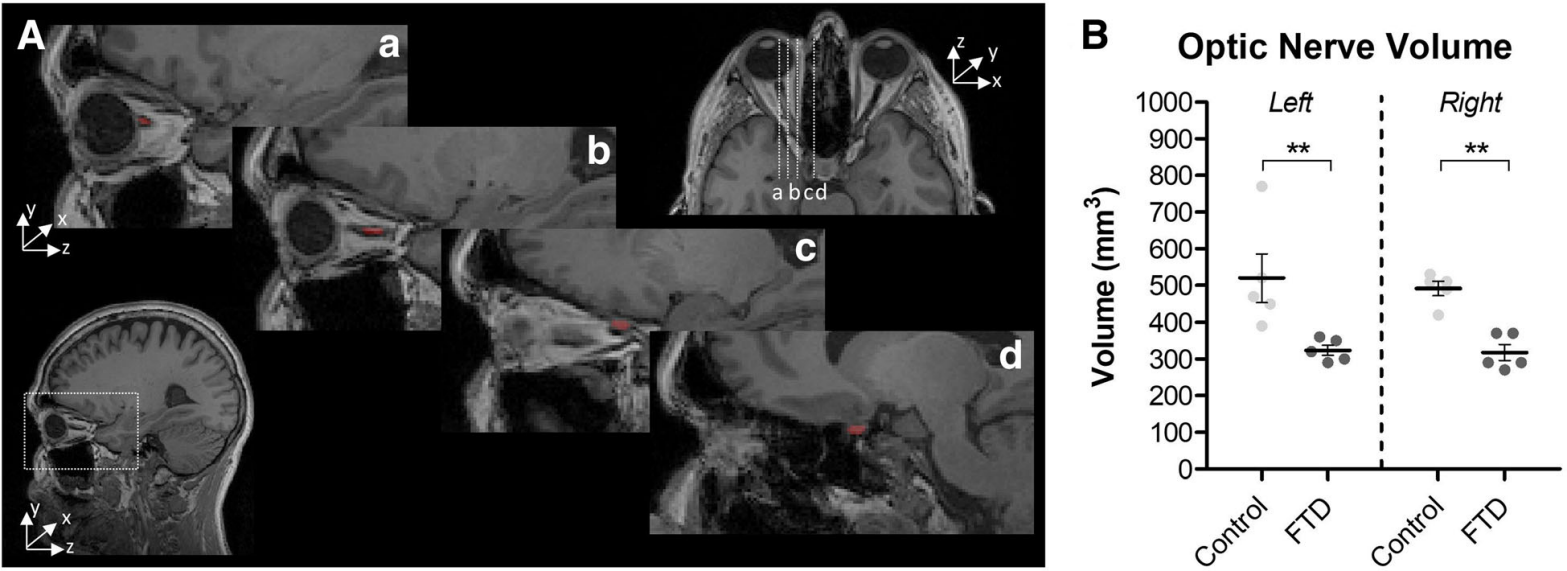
Reduced Optic Nerve Volume in MAPT Mutation Carrying FTD Patients. A Example images demonstrating the segmentation protocol used for volumetric analysis of the optic nerve from T1-weighted MR images. (a, b, c and d) Different sagittal levels of the 3D MR images, with the optic nerve highlighted in red. B Volume of the left and right optic nerves of FTD patients and age-matched healthy controls. Two-way ANOVA (*n* = 5, F_1,1_ = 25.57, *p* = 0.0001). Statistical significance indicated with asterisks:  = *p* < 0.01
